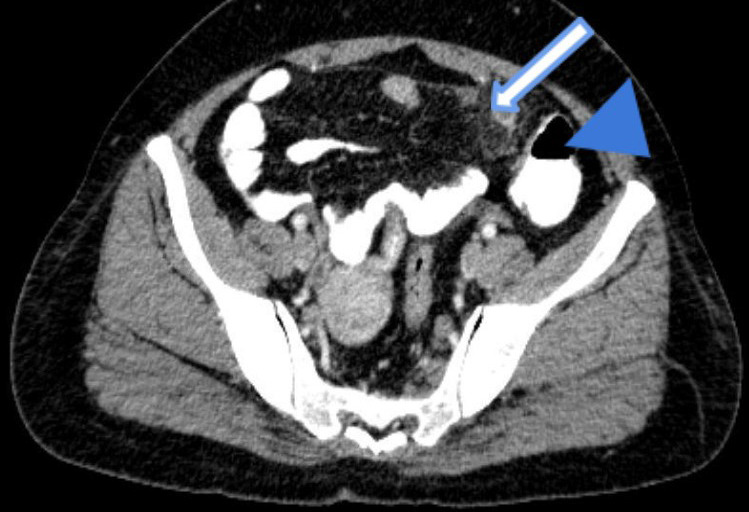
Axial image of contrast-enhanced CT scan of abdomen and pelvis shows inflamed appendix (block arrow) arising from the caecum (arrowhead) in the left iliac fossa.
